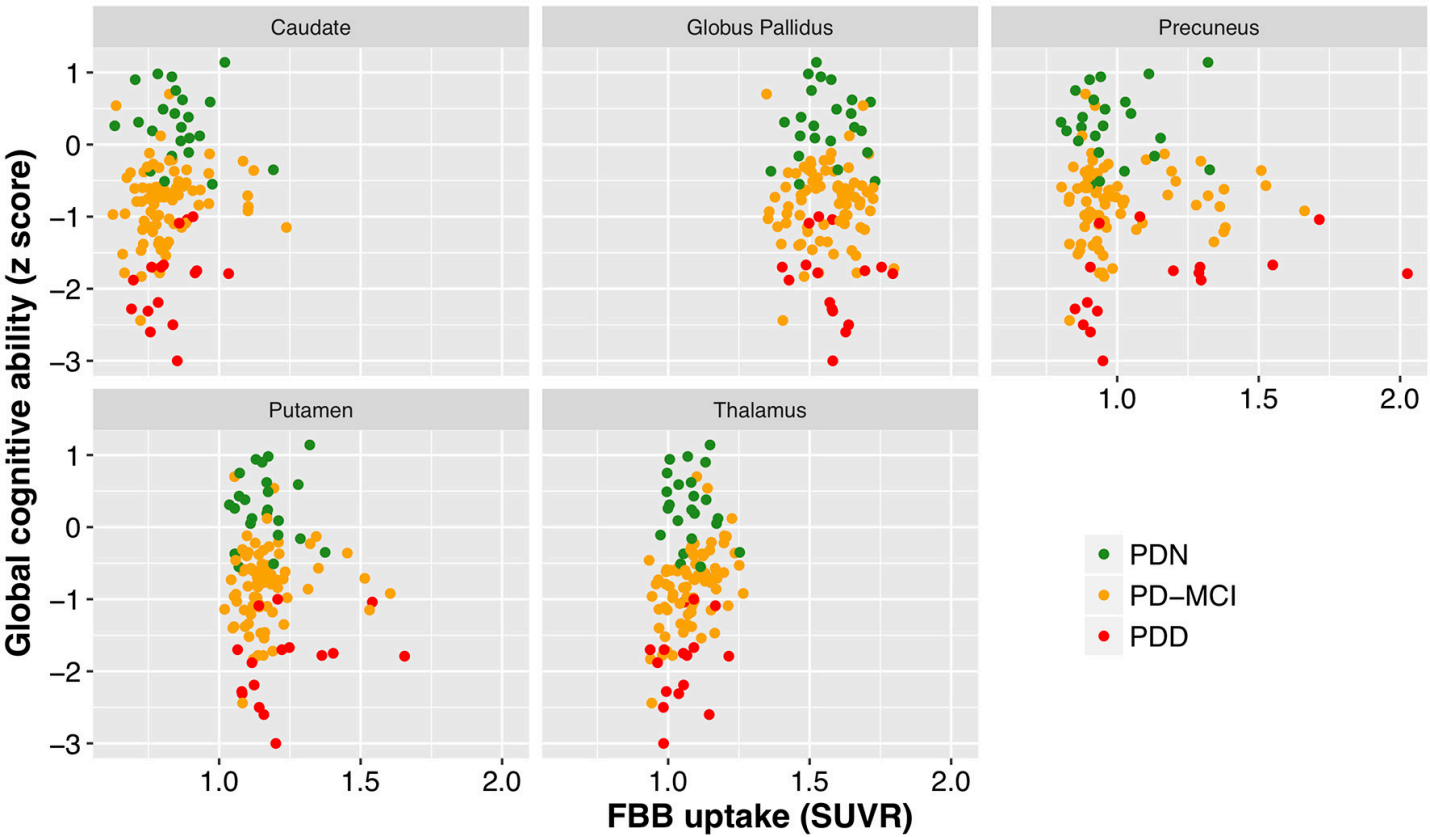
Cognitive performance as a function of mean standardized uptake volume ratios (Florbetaben) within a number of brain regions. While different regions exhibited different levels of amyloid deposition, there was a clear lack of relationship between cognitive performance (cognitive z score) and SUVR within all of the regions examined. FBB, Florbetaben; SUVR, standardized uptake volume ratio. Color represents cognitive status: green—Parkinson's with normal cognition (PDN), orange—Parkinson's with mild cognitive impairment (PD-MCI), red—Parkinson's with dementia (PDD).
